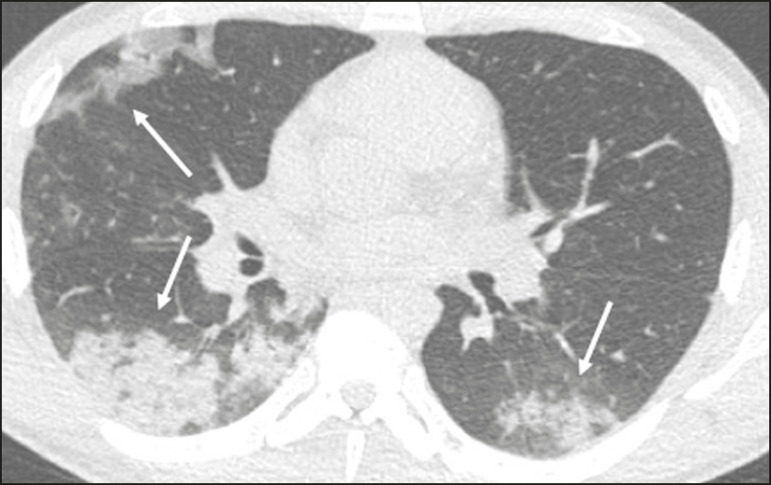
Chest CT scan of a 63-year-old male patient with a 7-day history of dyspnea and episodes of fever who tested positive for SARS-CoV-2 on RTPCR, showing bilateral peripheral pulmonary consolidations (arrows).
